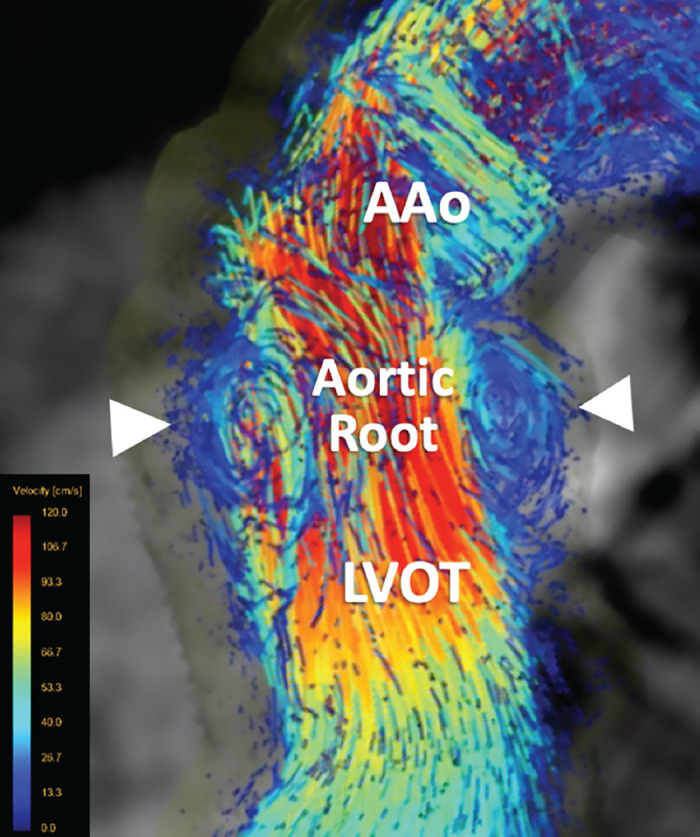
Four-dimensional (4-D) flow MRI image showing the physiological recirculating vortical flow in the aortic root of a healthy subject. Adapted from Ref. 159, with permission per Open Access terms. The white arrowheads show the vortices in the sinuses of Valsalva. AAo, ascending aorta; LVOT, left ventricular outflow tract.
